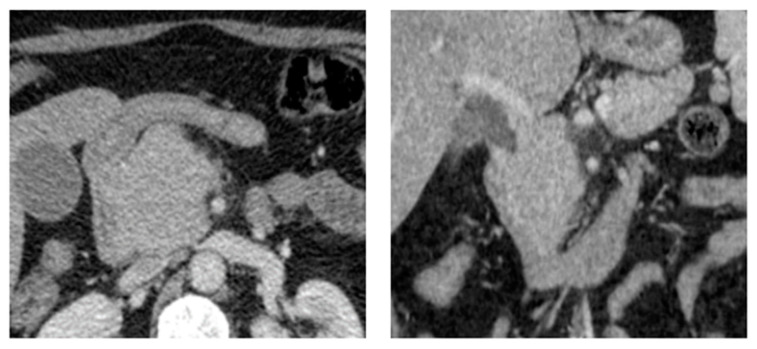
Biopsy-proven Pancreas EP, case from our institution. Axial and coronal post-contrast CT shows a well-defined homogenous mass at the pancreatic head. The coronal imaging demonstrates associated dilatation of the common bile duct.
